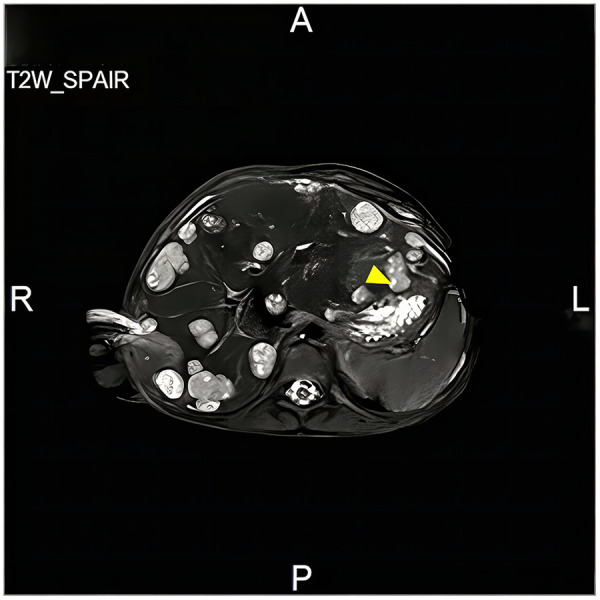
The patient's abdominal MRI, T2W_SPAIR sequence, demonstrates multiple hyperintense masses within the liver.
